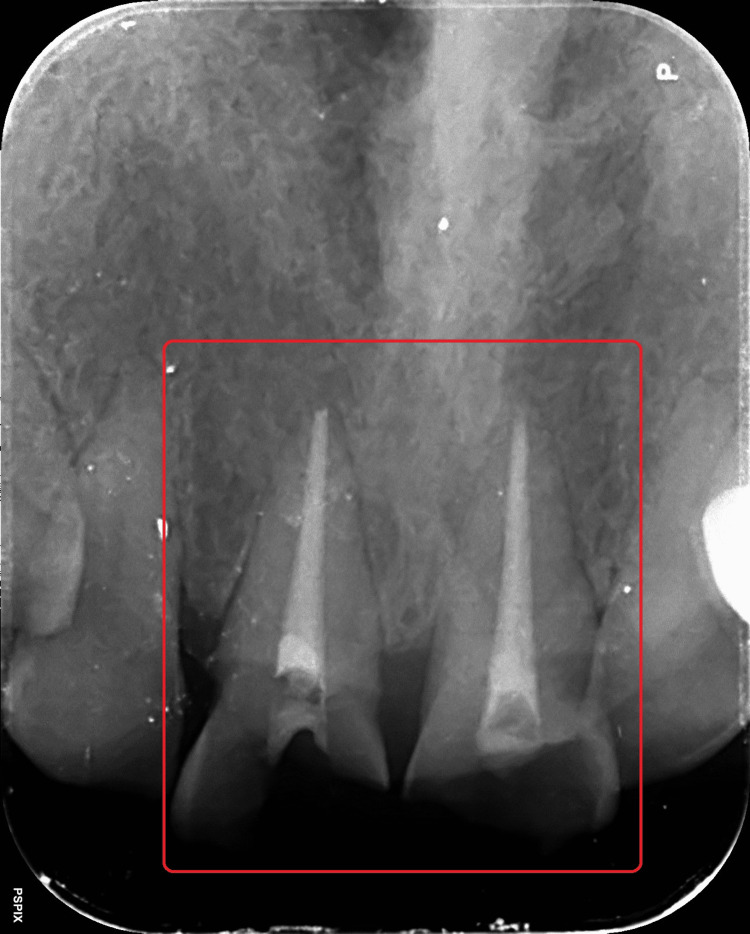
Diagnostic IOPA with respect to teeth 11 and 21 IOPA: Intraoral Periapical Radiograph
